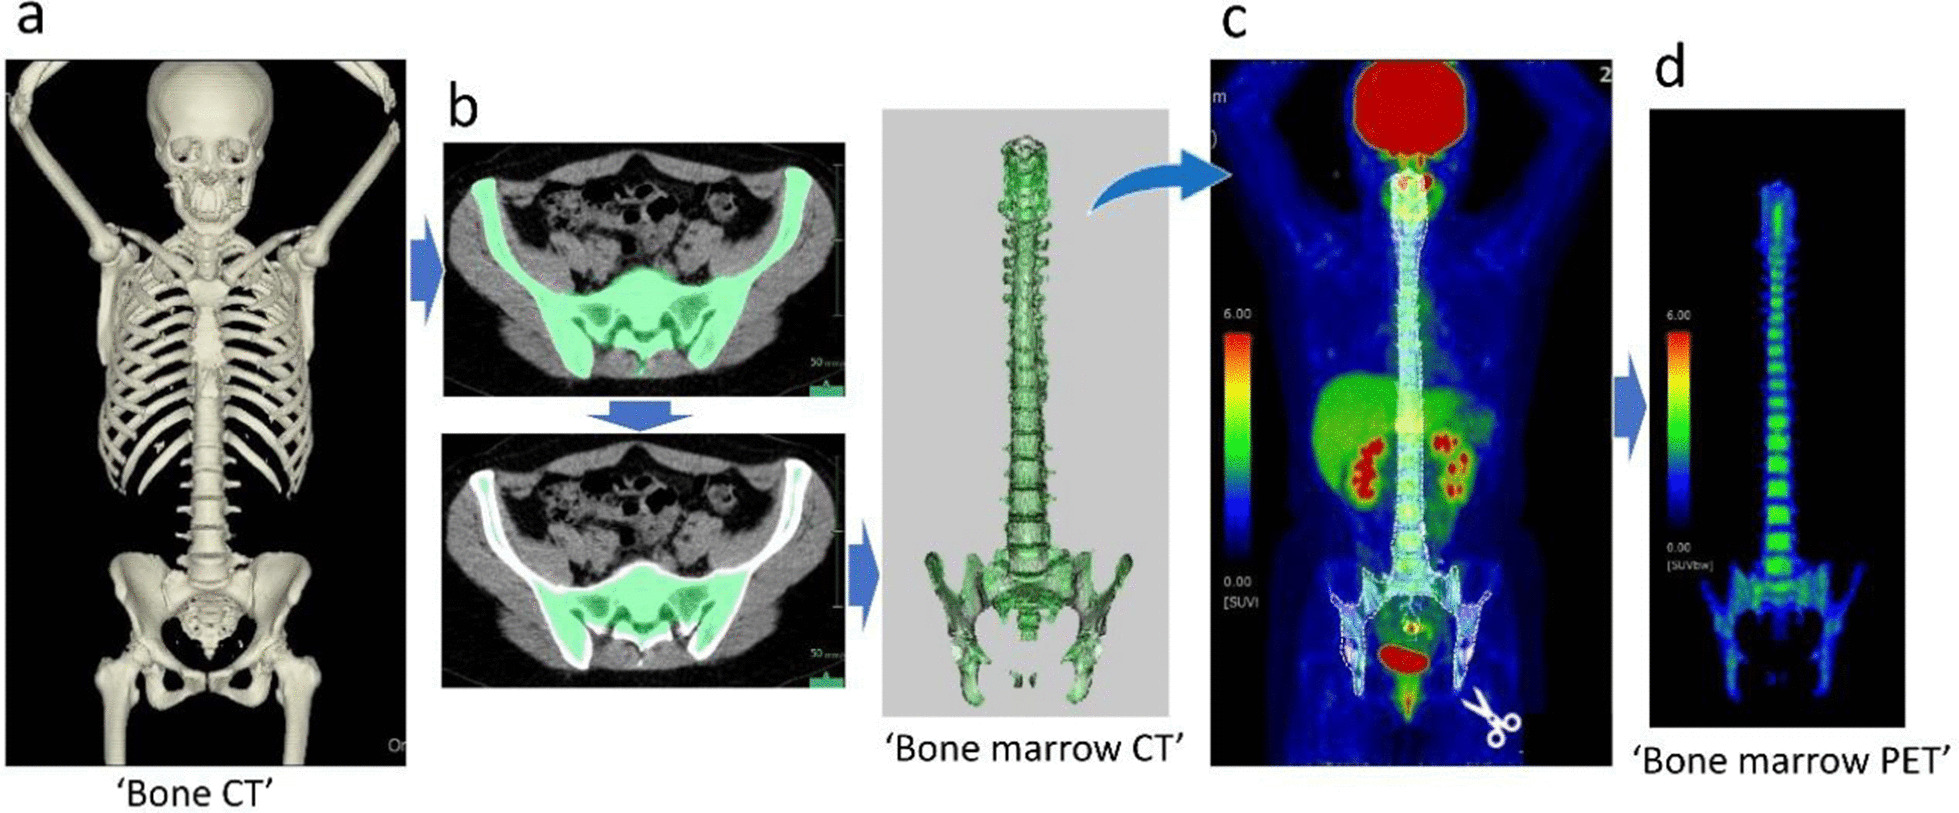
Two major procedures to obtain bone marrow (BM) positron emission tomography (PET). First: “BM computed tomography (CT)" was obtained. Whole spine and pelvic bones, including the BM, were automatically extracted from whole-body CT images using a bone-extraction algorithm implemented in the analyzer for automatic bone segmentation based on multi-task three-dimensional fully convolutional networks (3D-FCN) [19]. A multi-task 3D-FCN for organ segmentation has surpassed the two-dimensional FCN approach [20] by using data from multiple segmentation datasets and showed improvement over the single-task 3D-FCN approach (a). The contour lines were subsequently set to 2 pixels inside the first line, which were able to extract the BM and remove as much cortical bone as possible (b). Second: overlay bone marrow CT on whole-body PET (c), and obtain “bone marrow PET” masked with bone marrow CT (d)
